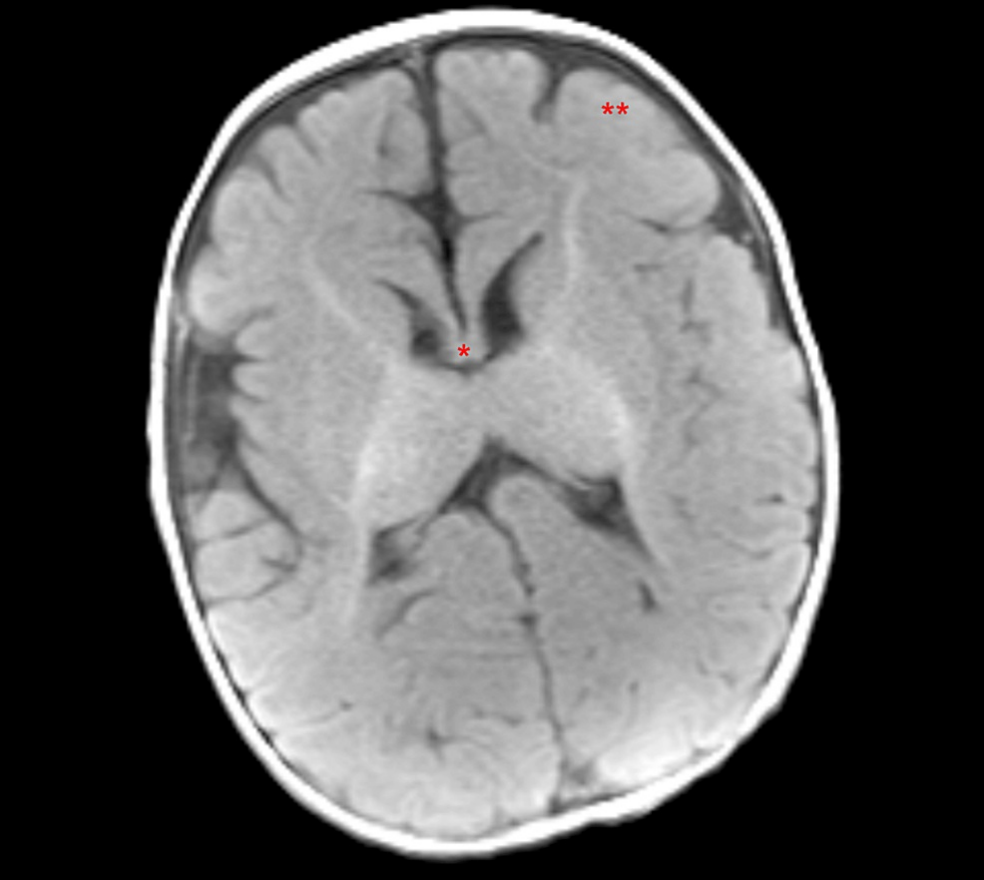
Patient's brain MRI showing corpus callosum hypoplasia and cortical malformations in the frontal-parietal lobes, suggestive of pachygyria * Corpus callosum hypoplasia  Pachygyria
